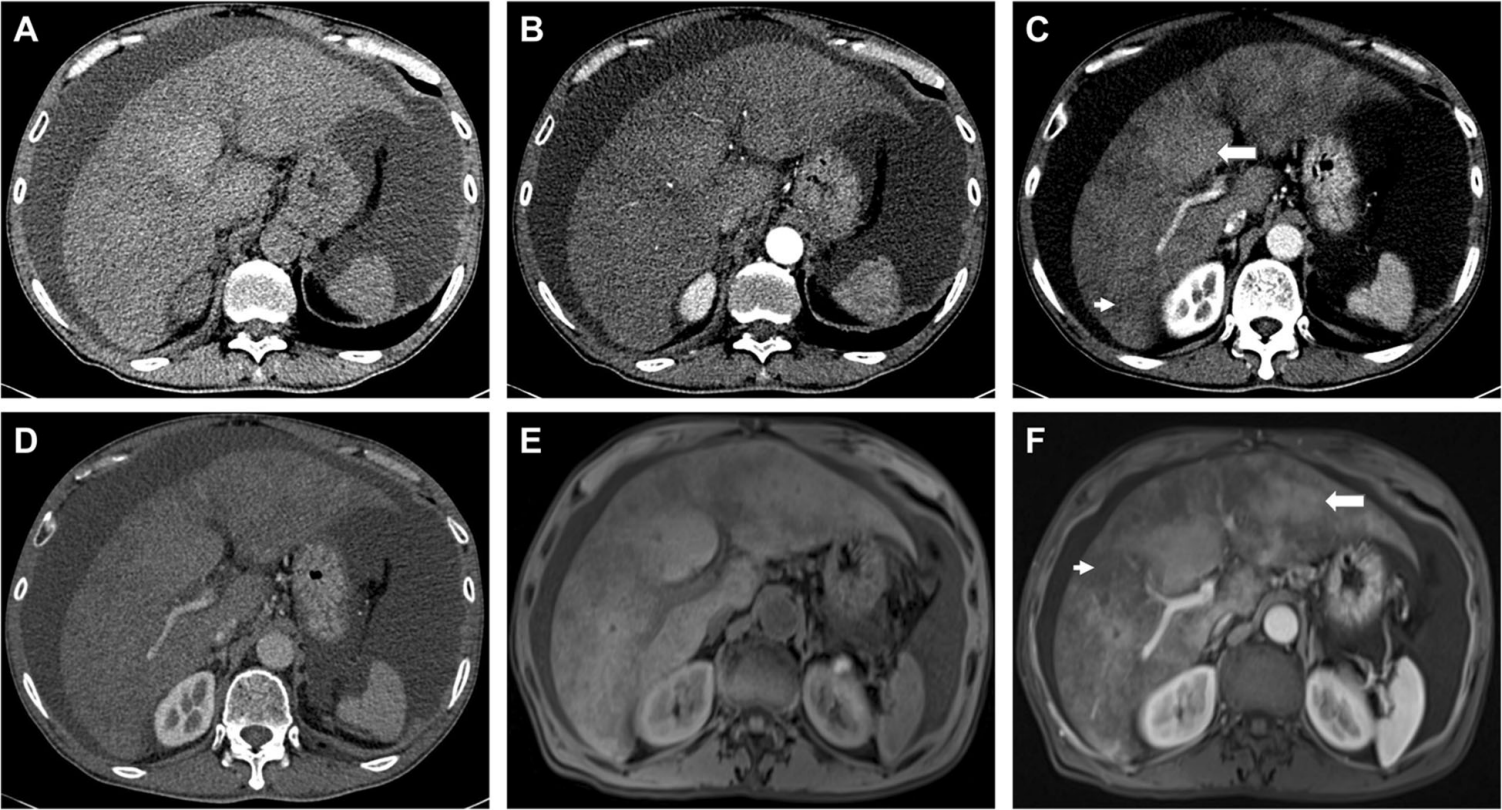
Elderly male patients diagnosed with gynura segetum-induced HSOS received contrast-enhanced CT and MRI scan. a-d images of plain and contrast-enhanced CT scan; a plain CT scan; b arterial phase; c porta-venous phase; patchy liver enhancement (arrow) and heterogeneous hypoattenuation (arrowhead) were shown; d equilibrium phases. e-f: images of pre-contrast and portal-venous phase on dynamic contrast-enhanced MRI scan;e pre-contrast MRI scan; f portal-venous phase of MRI scan. Heterogeneous hypointensity (arrowhead) and patchy enhancement (arrow) were shown
